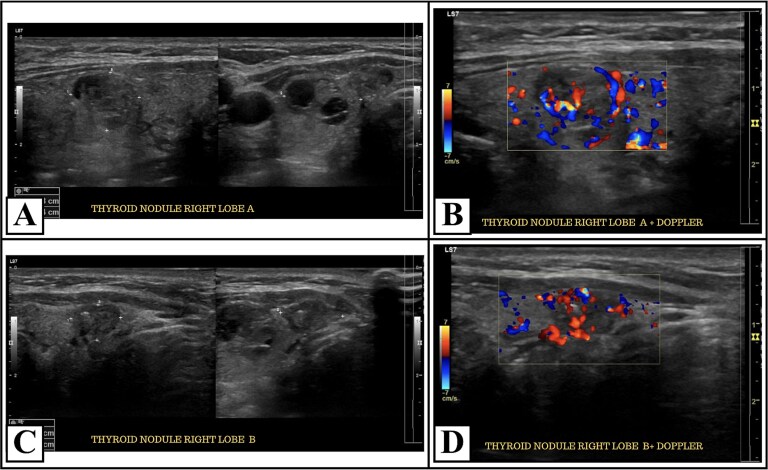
Thyroid ultrasound and doppler findings of right lobe nodules. Panel (A) shows a hypoechoic micronodule measuring 12 × 14 × 10 mm, with an irregular margin and a taller-than-wide configuration. Panel (B) shows color Doppler ultrasound demonstrating vascular flow within the hypoechoic right thyroid nodule. Panel (C) shows a solid hypoechoic nodule measuring 9 × 11 × 6 mm, with irregular margins and taller-than-wide shape. Panel (D) demonstrates color Doppler ultrasound of the solid right thyroid nodule; showing intranodular vascularity.
